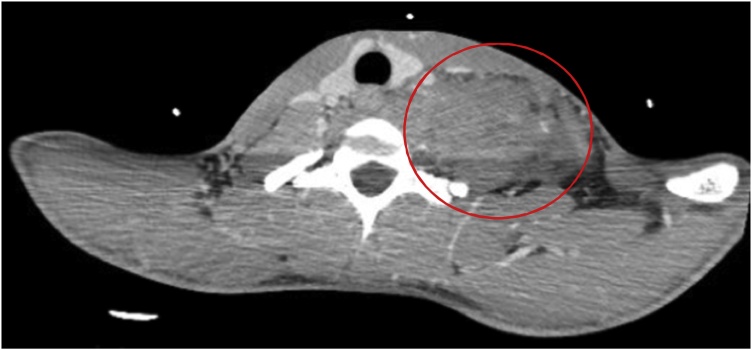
CT Angiography Chest. A large, rapidly expanding hematoma creating an asymmetrical enlargement with slight deviation of the trachea to the right – away from the hematoma. Subcutaneous emphysema is noted in this image as well.
